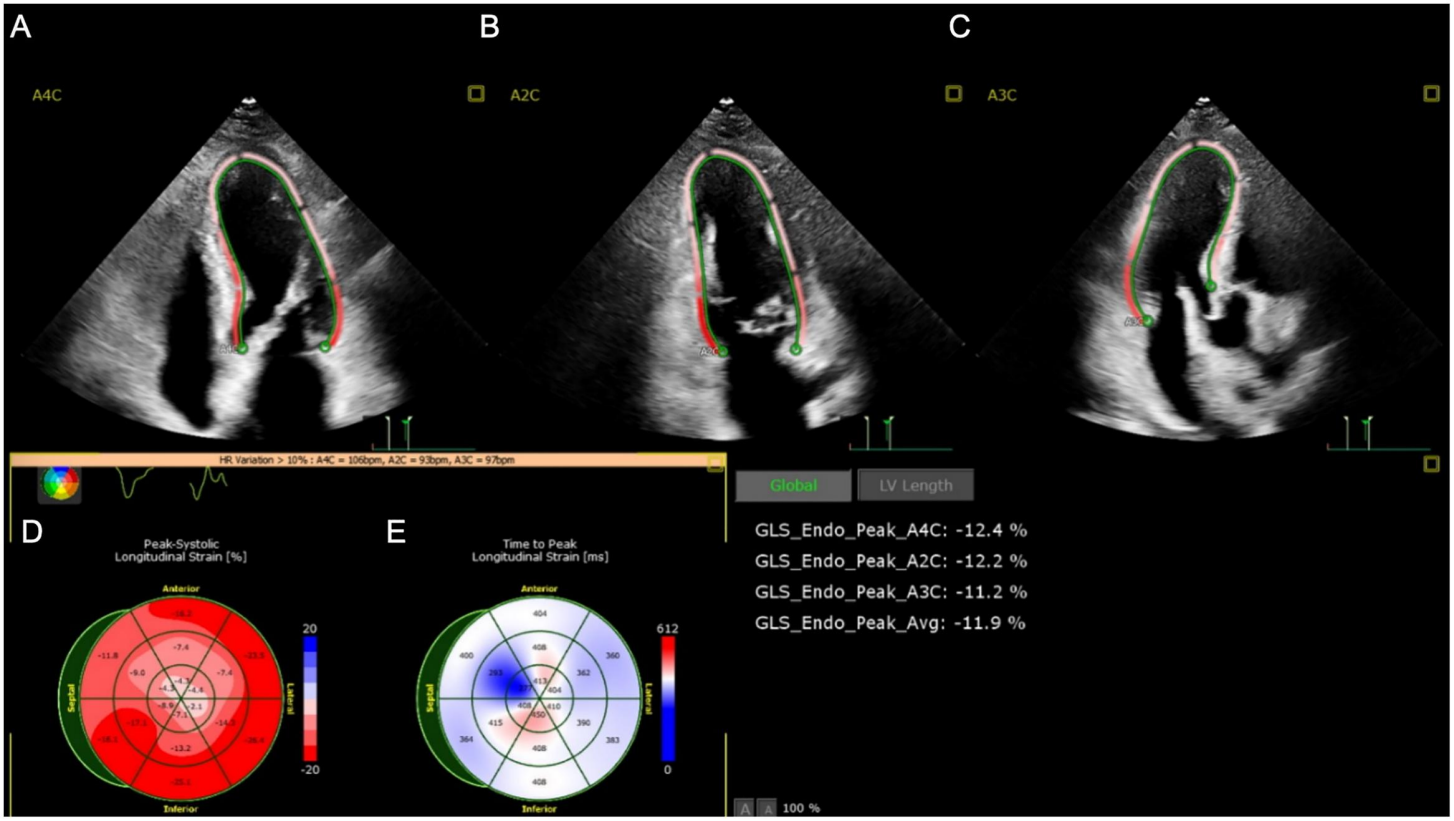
The results of the patient's echocardiogram. (A,B,C) Ultrasound images of the patient's heart in the 4-chamber, 2-chamber, and 3-chamber views. (D,E) Graph of the Peak-Systolic Longitudinal Strain of the heart.
